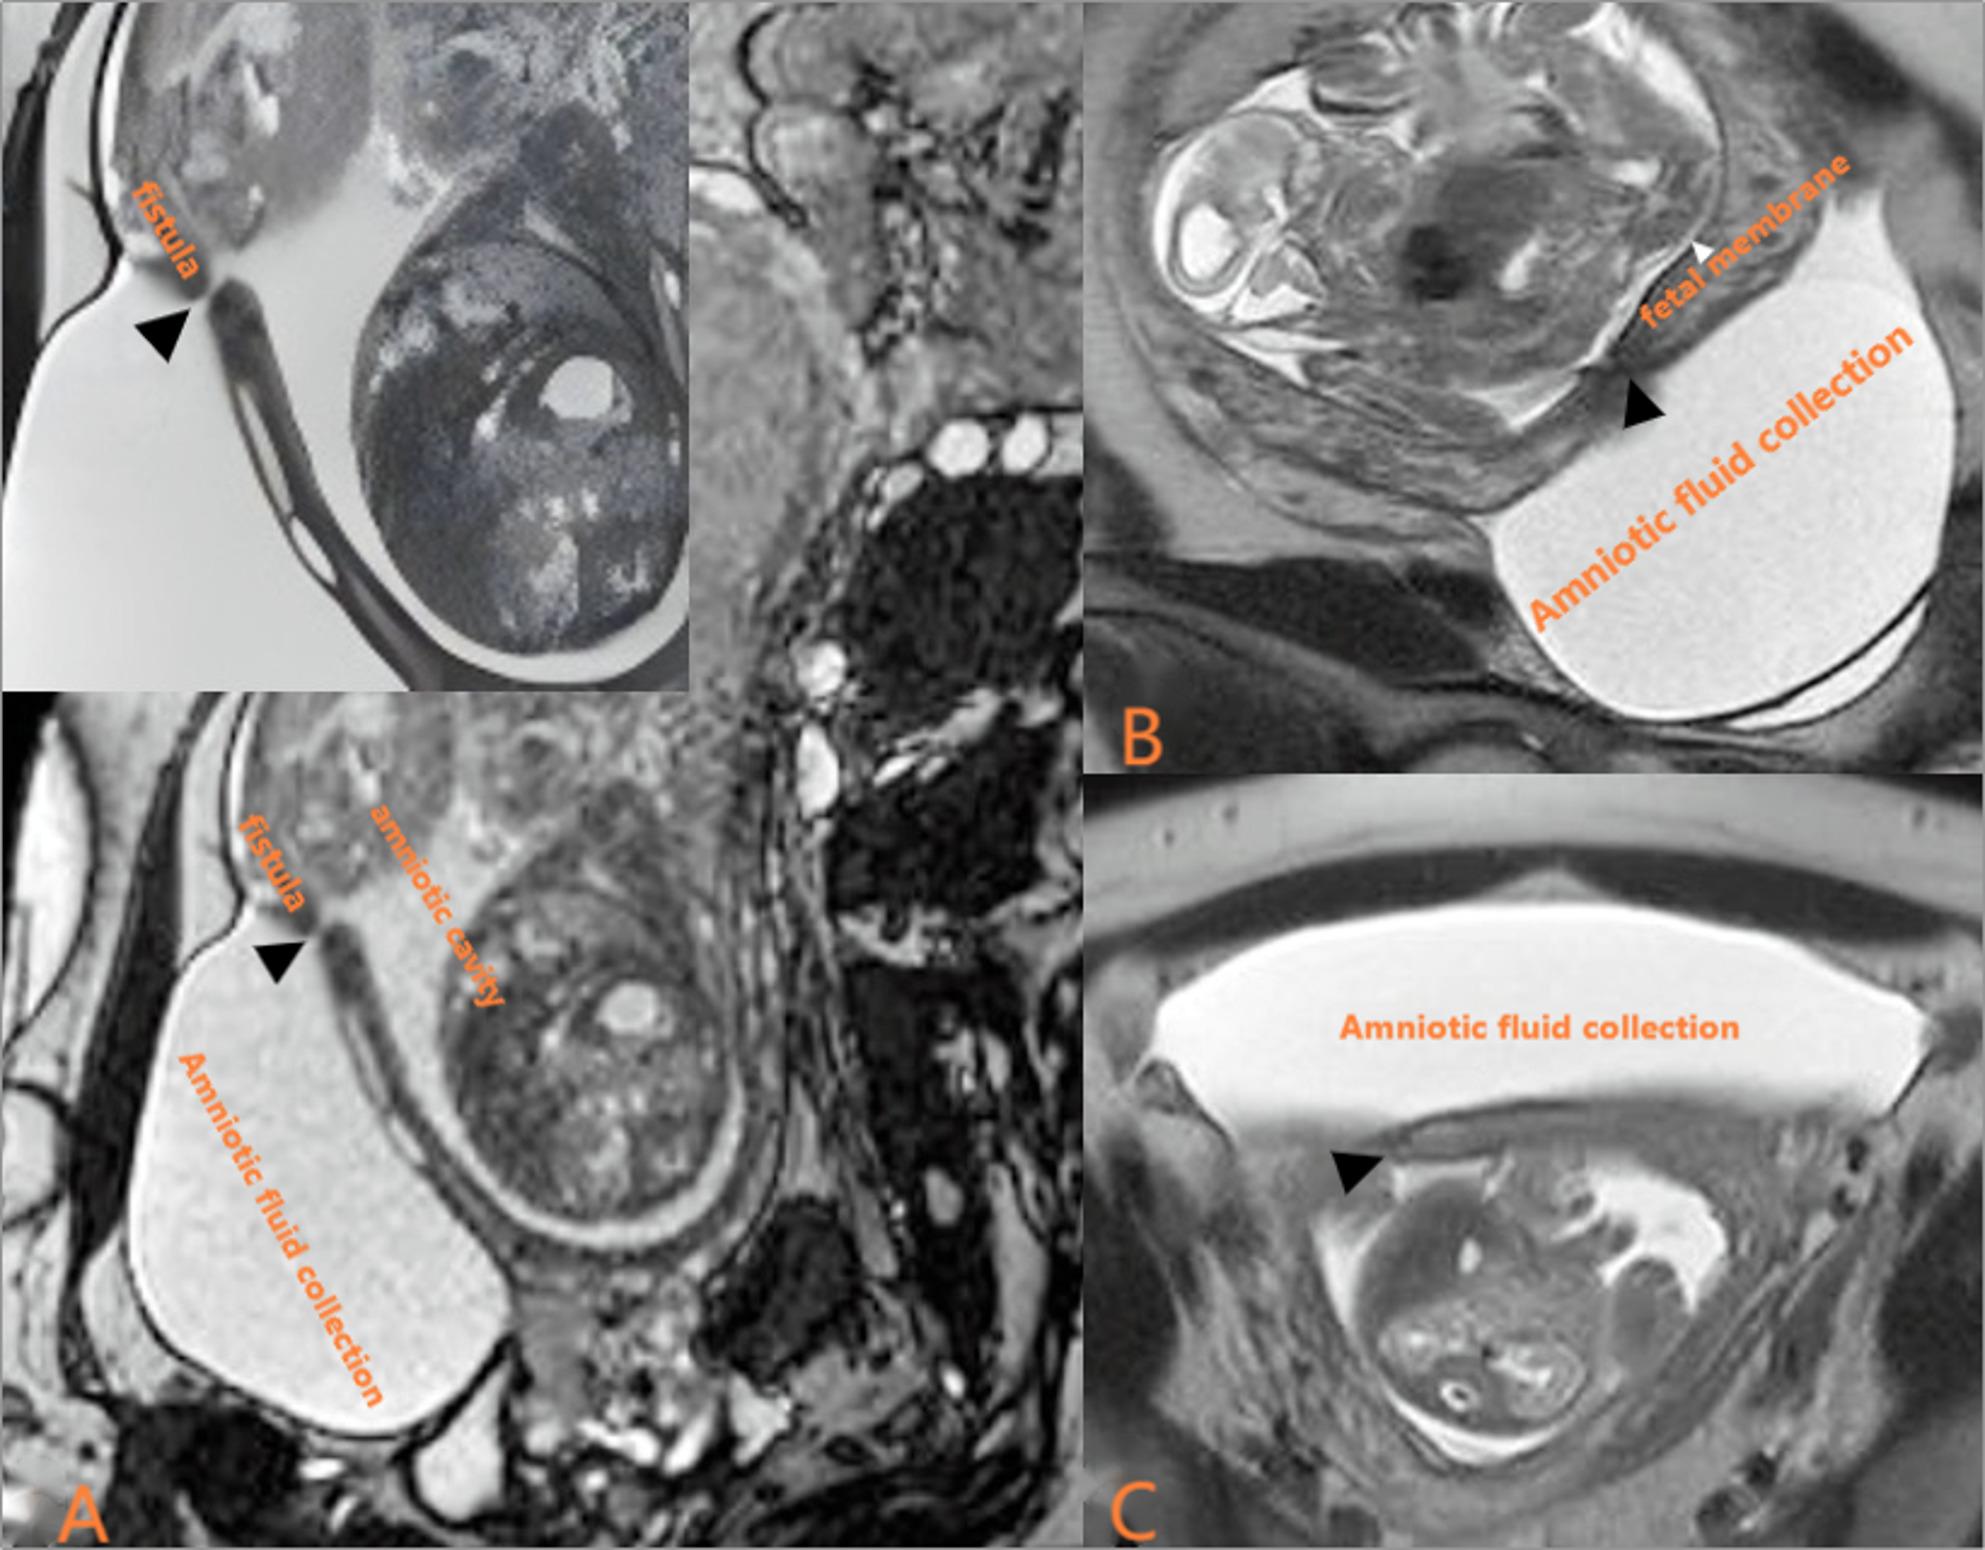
MRI at 20 weeks of gestation. A Oblique sagittal BSSFP image shows a fistulous tract (black arrow) through the anterior uterine wall at the previous puncture site, with localized fluid in the vesicouterine pouch compressing the bladder. Intra amniotic fluid is reduced. B Coronal T2WI reveals undulating, collapsed fetal membranes (white arrows). C Axial T2WI confirms the fistulous tract (black arrow) and the fluid collection
